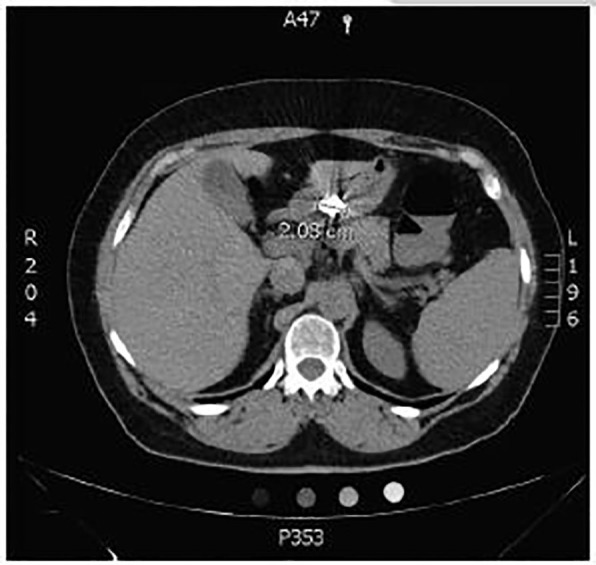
Axial view from computed tomography of abdomen and pelvis showing a 2cm foreign body in the stomach.
